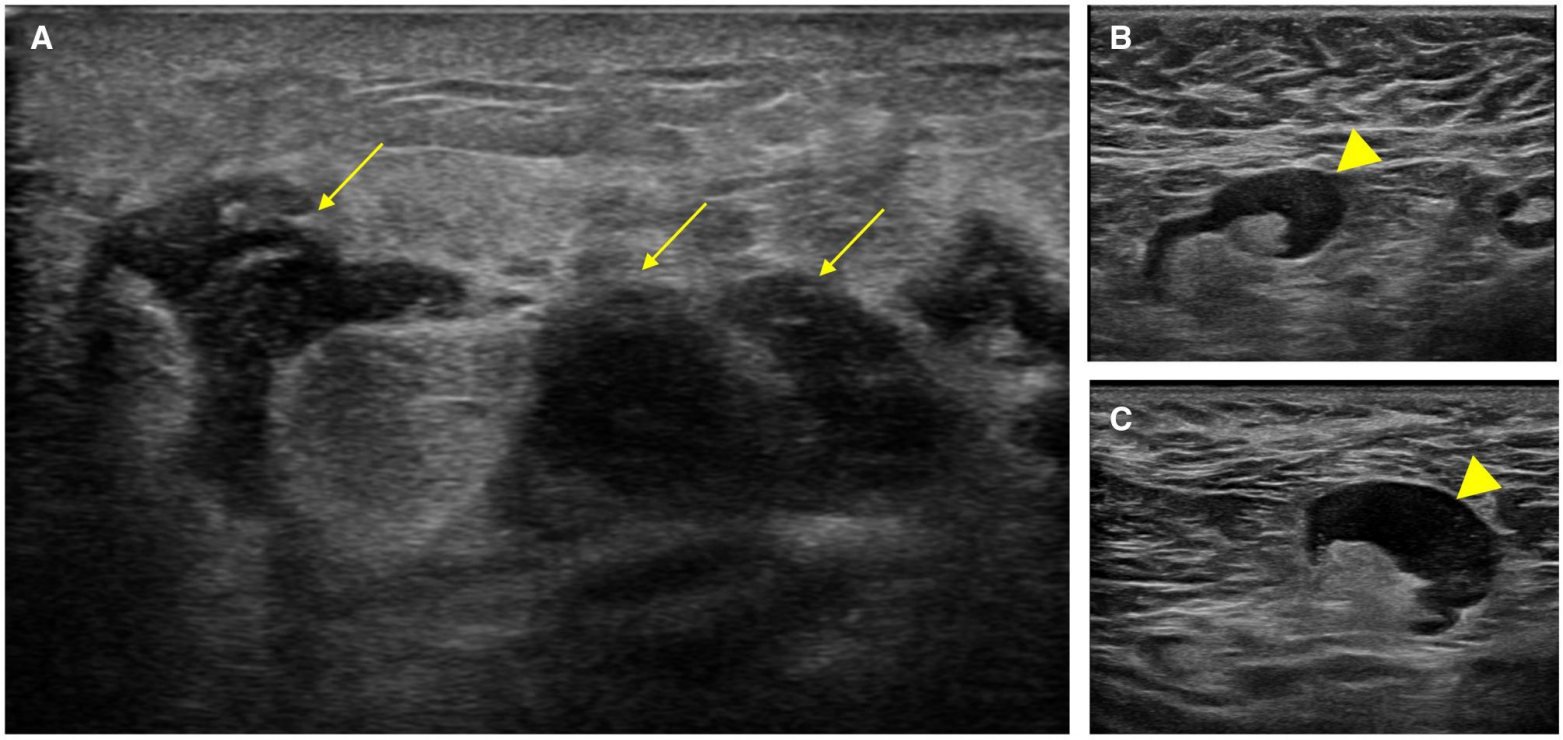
Ultrasound of the breast and axilla. (A) Underlying the palpable mass in the left breast, there is a large ill-defined heterogeneous zone with multiple irregular hypoechogenic areas and posterior acoustic shadow (arrows). (B, C) Multiple adenopathies with focal cortical thickening are seen in level I of the ipsilateral axillary lymph node chain (arrowheads).
